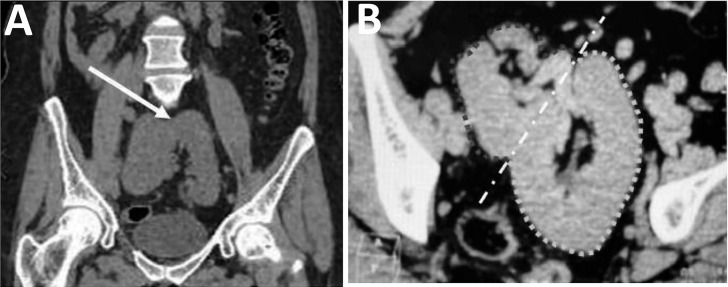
Renal assessment: (A) Coronal CT scan showing a renal ectopia together with an abnormal rotation of the kidney, with apparent fusion of the kidney in the pelvis, (B) Axial CT scan clearly showing the renal ectopia, with the lobulated kidney occupying an abnormal position in the pelvis. The fusion of the kidney is confirmed in this section, where the anteriorly directed hilum is observed.
